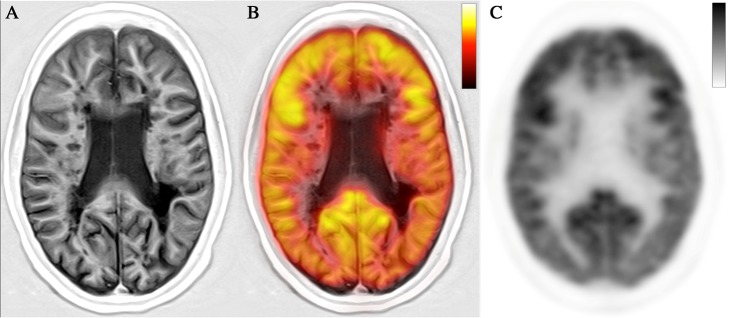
Synchronous PET/MRI acquisition in a RRMS female patient. ^18^F-FDG PET/MRI acquired using Siemens Biograph mMR (University-Hospital of Padova): a Phase Sensitive Inversion Recovery (PSIR) sequence showing small multifocal WM lesions in a RRMS female patient. b fused ^18^F-FDG PET and PSIR sequence. c) ^18^F-FDG PET showing that WM lesions are frequently isometabolic to the WM
